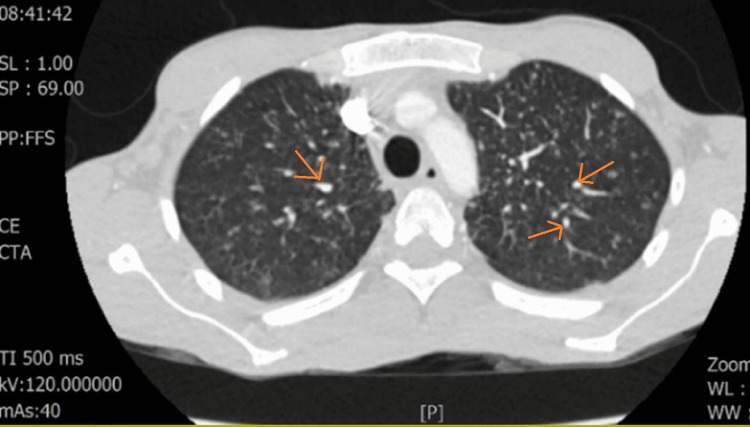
Lung window view demonstrating the bilateral pulmonary nodules indicated with arrows on the patient's chest CT.
